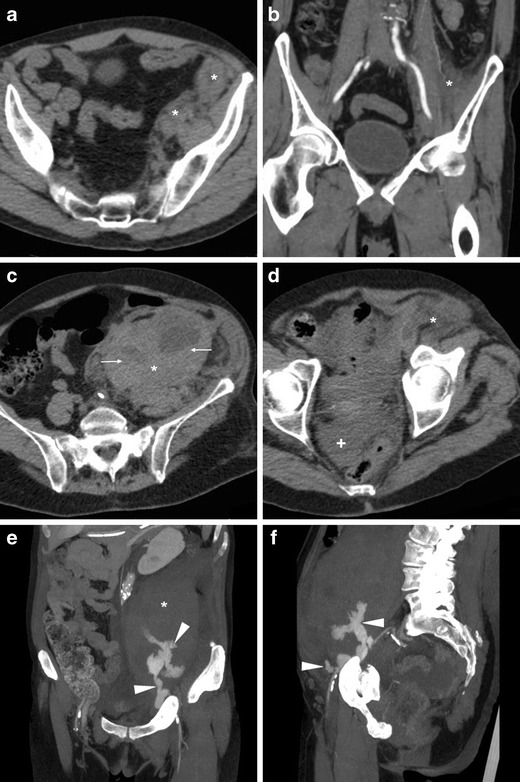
Intra-abdominal haemorrhages. A-B) In a 66-year-old man with severe peripheral artery disease (PA), shortly after percutaneous transluminal angioplasty (PTA) and stenting of the left femoral artery with ipsilateral vascular access, precontrast (A) CT images showed high-attenuation subperitoneal effusion (*) along the left iliac muscle, without active bleeding at CT-angiography (B), which was treated conservatively. C-F) In a 75-year-old woman with obliterating PAD, following PTA and stenting of the right superficial femoral artery via left CFA access, precontrast images (C, D) showed extensive, mass-like left retroperitoneal haematoma (*) with characteristic fluid-fluid levels (thin arrows), mild haemoperitoneum (+) in the pelvic cul-de-sac. CT angiography with maximum-intensity projection (MIP) images (E, F) showed extraluminal CM flowing from the site of arterial injury, indicative of active bleeding. Note displaced ipsilateral kidney. Emergency surgery including retroperitoneal decaillotage was required to seal the leaking femoral artery
